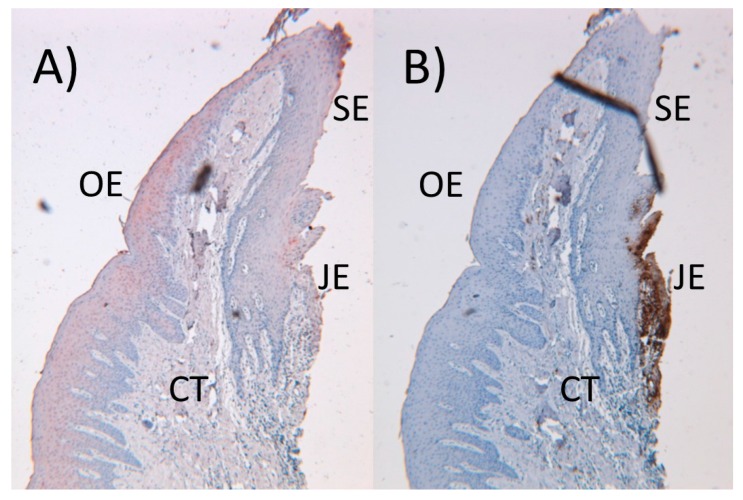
In a healthy gingiva, epithelial defensins (human β-defensins (hBD-2) in red color) are located in the oral (OE) and sulcular (SE) epithelia (A), while neutrophilic antimicrobial peptides (α-defensins in brown color) are located in the junctional epithelium (JE) and partly in connective tissue (CT) (B). (An original figure by U.K.G.)
